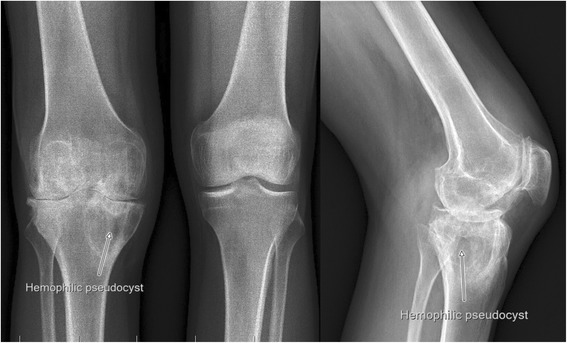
Preoperative X-ray of the typical hemophiliac arthropathy of the right knee with hemophiliac pseudocyst of the proximal tibia
